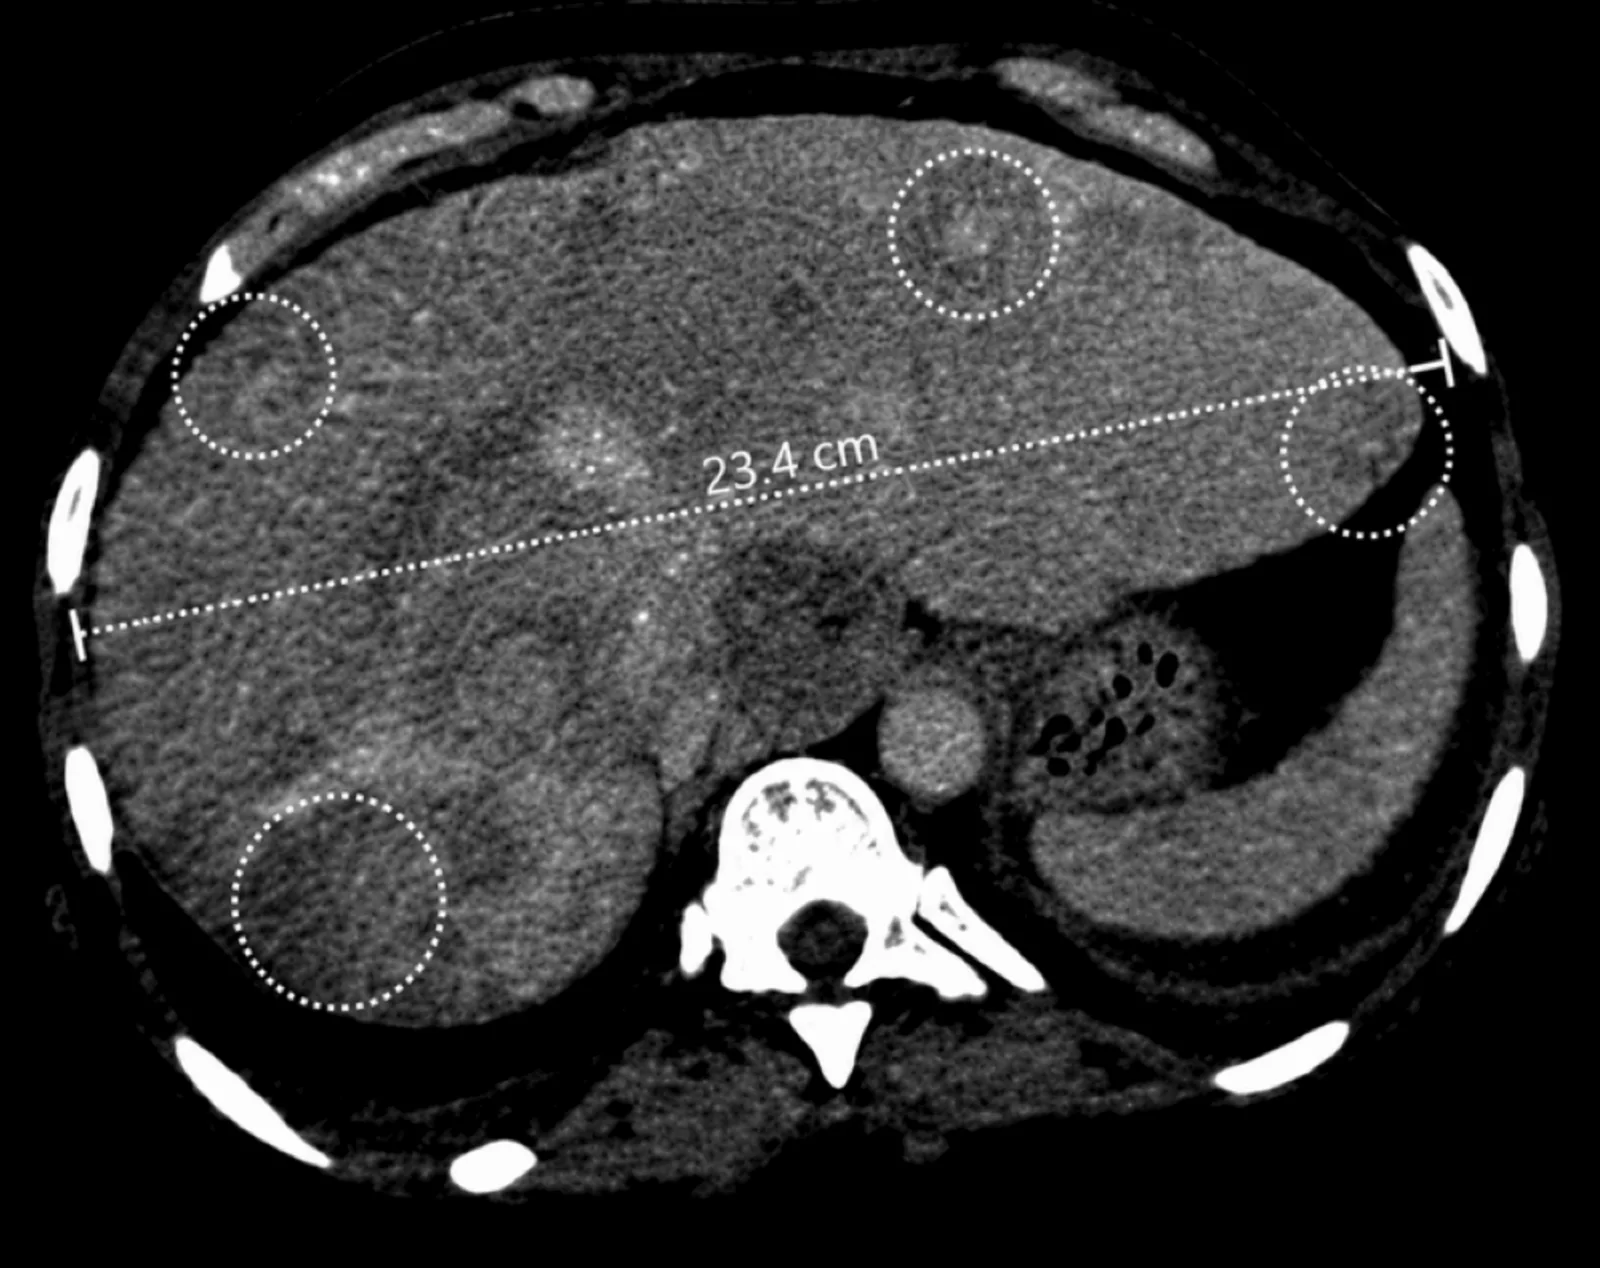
Contrast-enhanced axial CT of the abdomen demonstrating marked hepatomegaly (liver span, 23.4 cm). White dotted circles highlight multiple hypervascular hepatic lesions consistent with metastatic disease.
